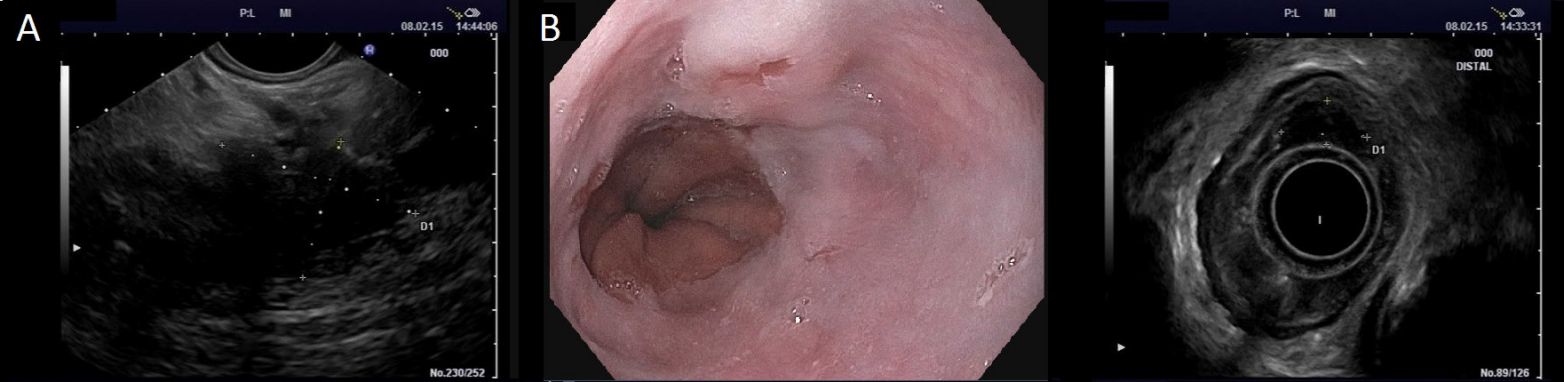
Visualization of the A. pancreatic lesion on endoscopic ultrasound (EUS) and B. esophageal lesion on endoscopy and EUS at the time of fiducial placement prior to SBRT
